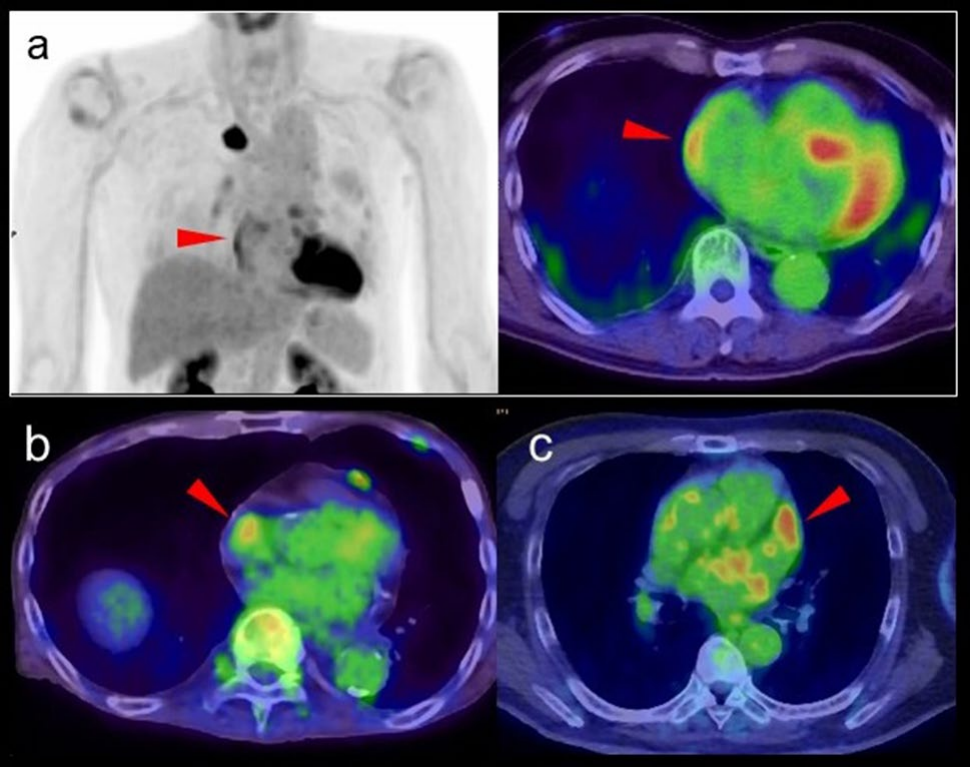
FDG uptake pattern associated with atrial fibrillation. Increased FDG activity along with the atrial wall (a red arrowheads), FDG uptake at right appendage (b arrowhead) and at left appendage (c arrowhead) are all associated with atrial fibrillation
